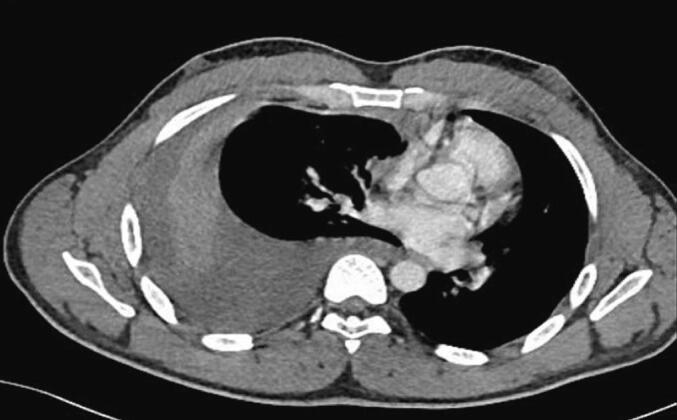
CT scan of the thorax demonstrating a massive right sided hemothorax with shift of the mediastinum to the left.
